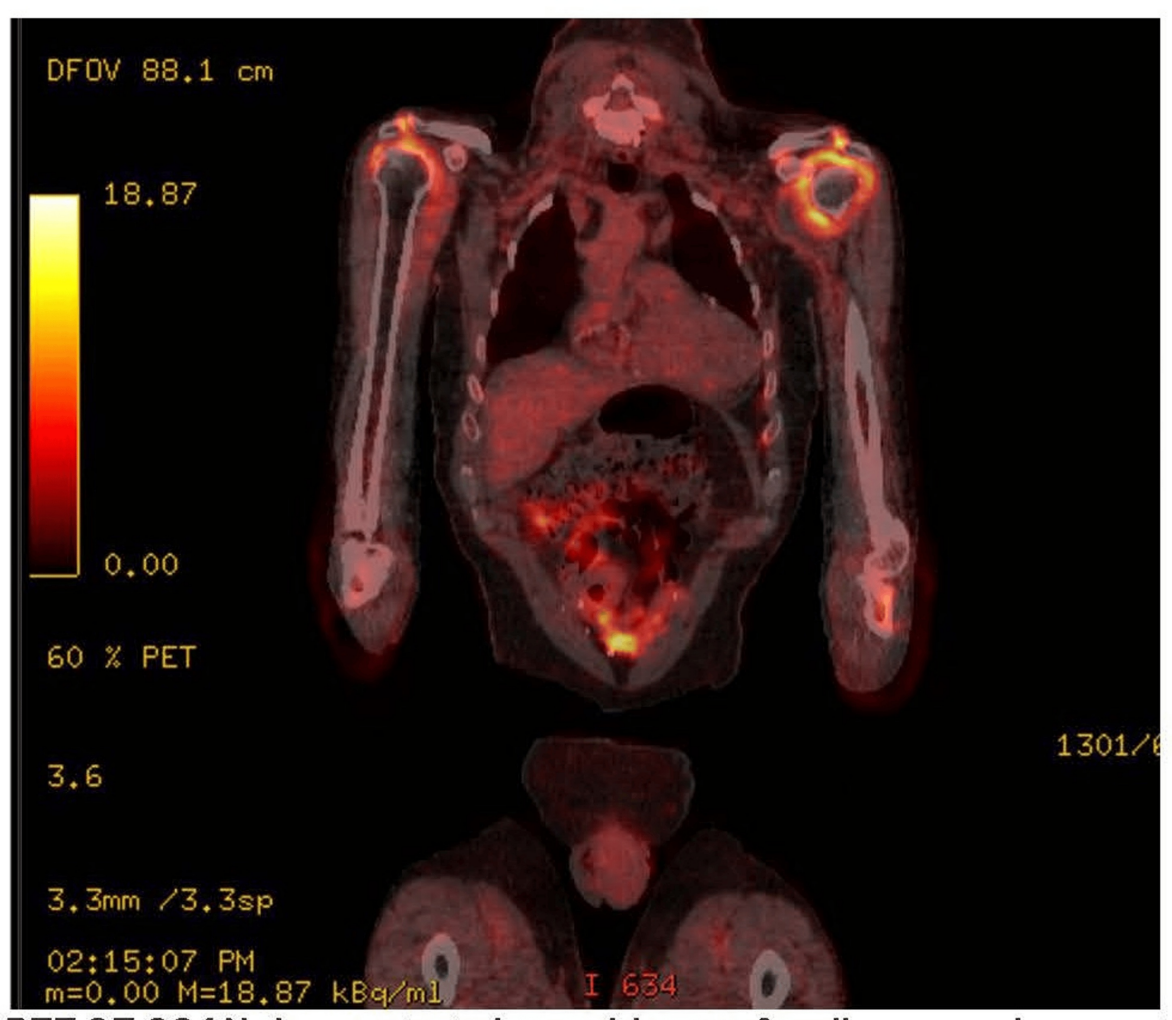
The PET CT demonstrated extensive symmetric periarticular fluorodeoxyglucose activity indicative of an active inflammatory process, with no evidence of focal destructive lesions, deep-seated infection, or vasculitis.
